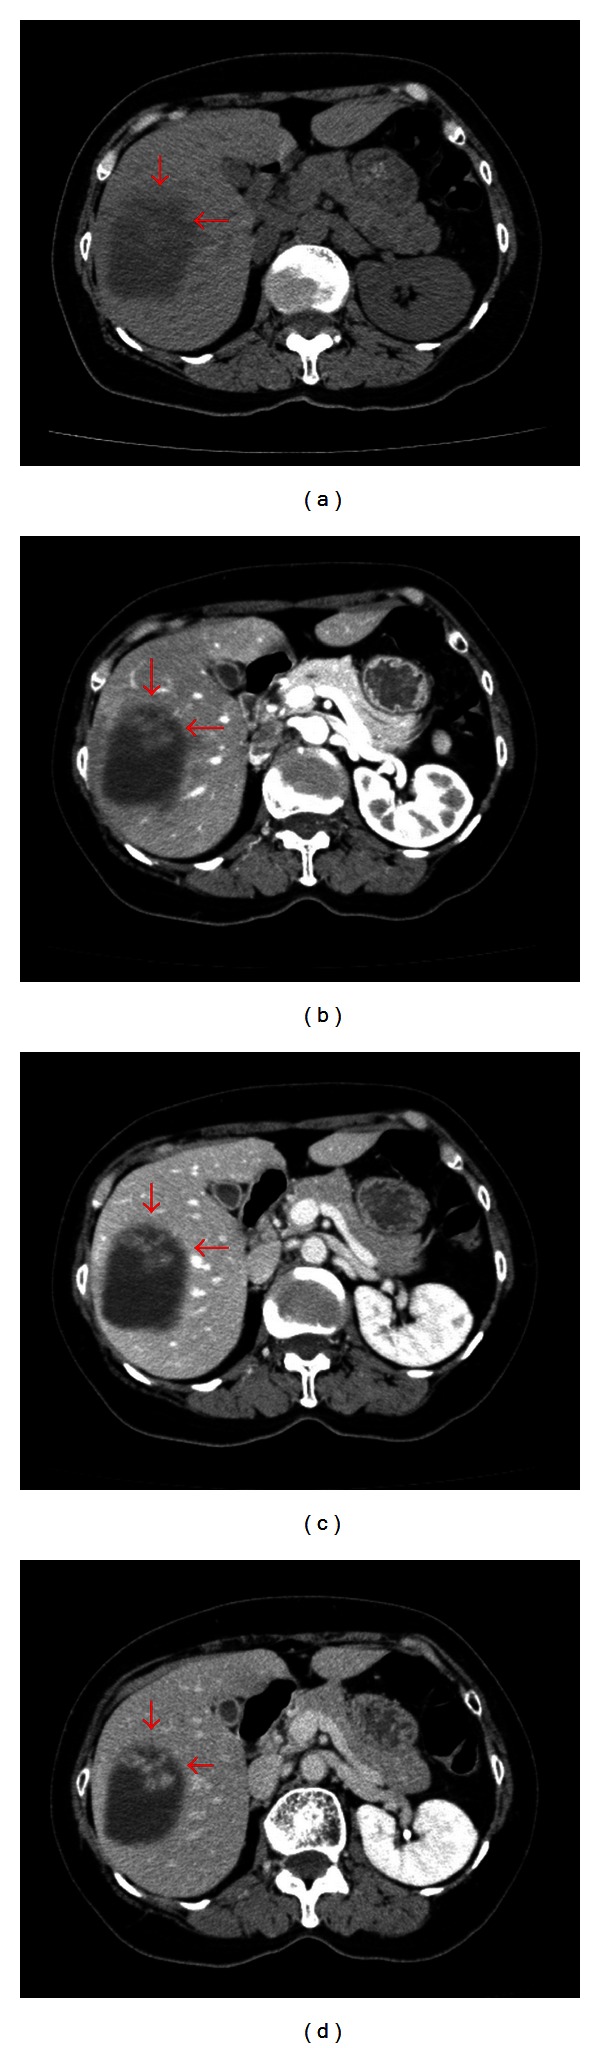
Dynamic contrast-enhanced computed tomography performed in February 2012. (a) Simple, (b) early phase, (c) portal phase, and (d) parallel phase images. The cystic mass occupying the right hepatic lobe was approximately 9.8 × 7.7 × 9.1 cm in size and had slightly increased in size since September 2011. Solid components exhibiting increased contrast uptake are observed at the base and left walls of the cyst (arrows).
